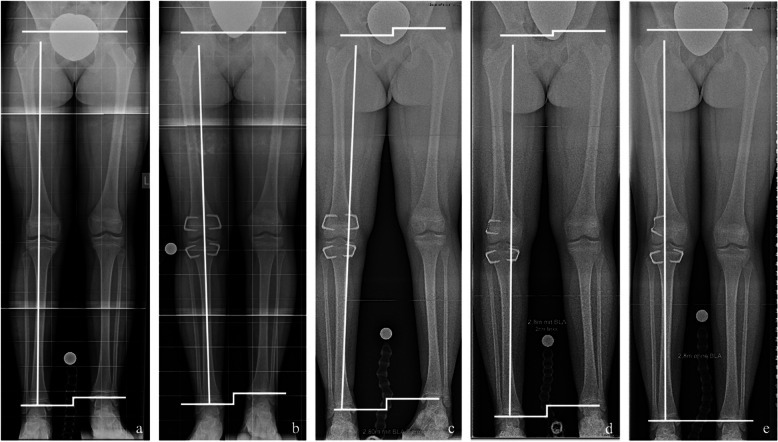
Secondary coronal angular deformity after temporary epiphysiodesis. a Anteroposterior long standing radiograph of a 10-year-old girl with predicted idiopathic leg length discrepancy (LLD) of 3 cm. b After temporary epiphysiodesis around the right knee using RigidTacks^TM^ (Merete, Berlin, Germany) showing neutral coronal alignment of the treated right leg. c After 16 months of treatment showing significant secondary femoral varus deformity of the right leg. d After conversion to a distal femoral hemiepiphysiodesis through implant removal on the concave side of the deformity and change to a flexible staple (FlexTack^TM^, Merete, Berlin, Germany). e After another 6 months of treatment showing restoration of the neutral coronal alignment of the right leg and complete LLD equalisation
